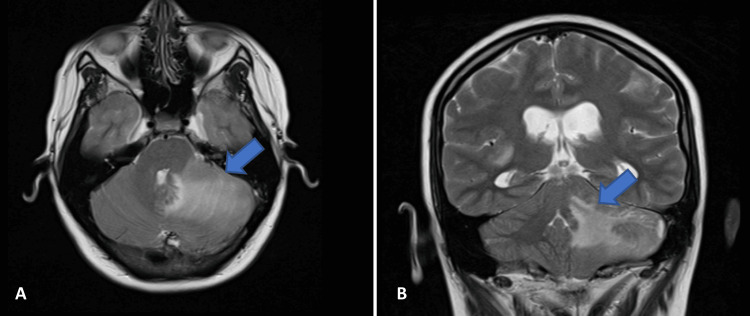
Brain MRI within the first few days after admission. The blue arrow (A, B) indicates the hypersignal in T2 FLAIR of the largest lesion in the left cerebellar hemisphere. Abbreviation: MRI, magnetic resonance imaging.
